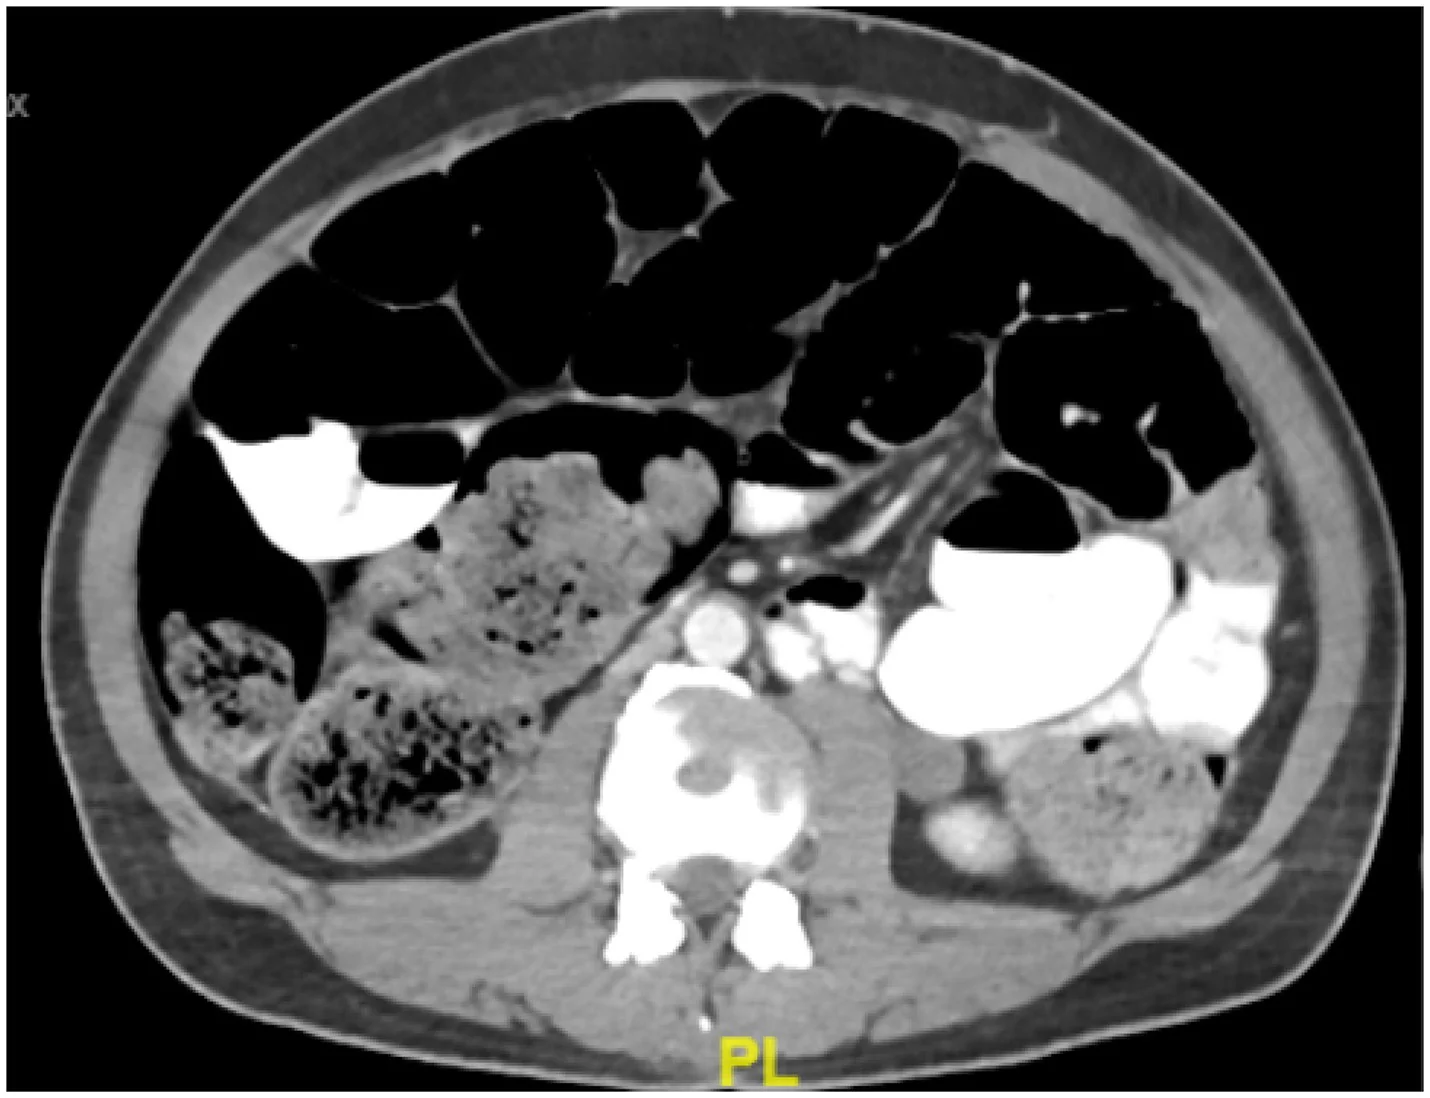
Contrast enhanced computed tomography (CECT) abdomen on 7th day of admission shows dilated bowels with stool impaction without significant small obstruction or perforation.
